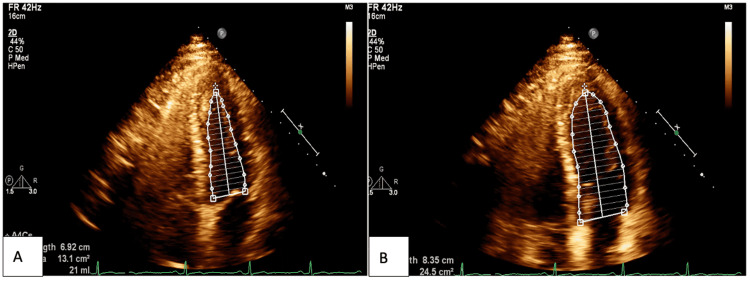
Apical four-chamber view of second follow-up echocardiogram after cardiac catheterization done after two weeks of treatment with colchicine A: Non-contrast study showing the left ventricle in systole; B: non-contrast study showing left ventricle in diastole. Note how there is now good contraction of all segments of the left ventricle when compared to Figure 2, indicating resolution of the apical hypokinesis. Ejection fraction improved to 60%.
